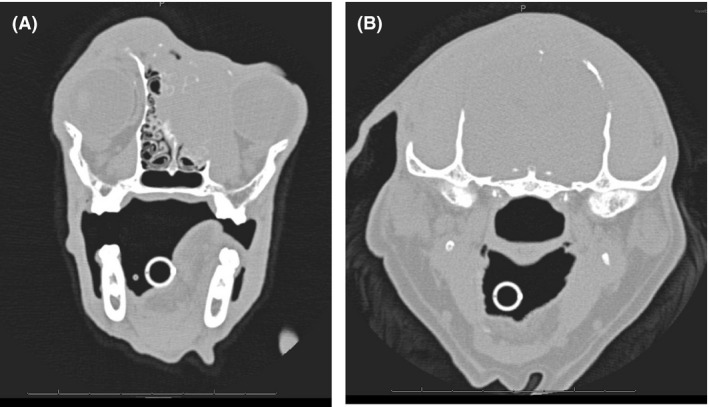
Computed tomography. Transversal plane. Five month after initiating chemotherapy. (A) Further progression of the mass with entering the orbit. (B) Destruction of the bone and suspected involvement of the brain.
